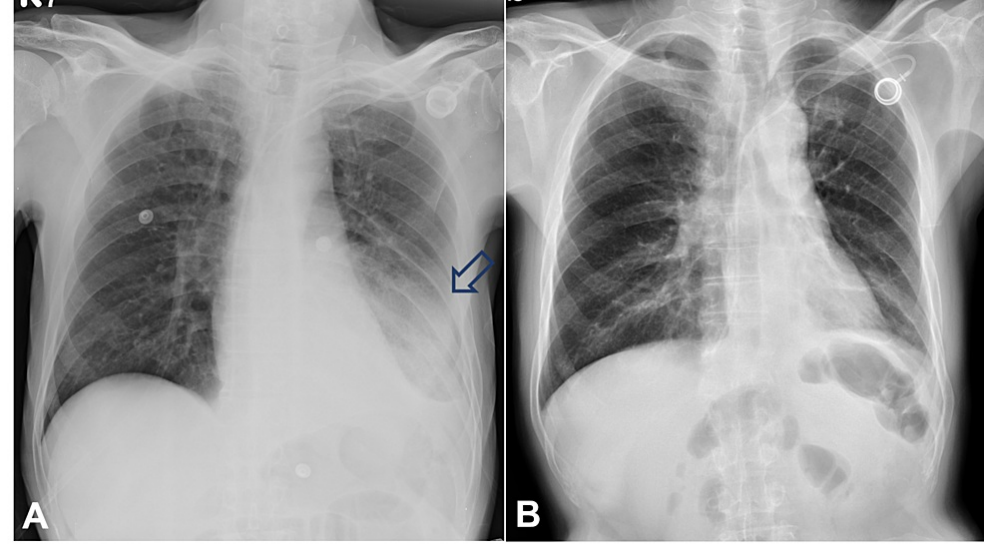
Chest X-ray films of the patient during treatment. A. Pneumonia in the left lower lobe (blue arrow) four days after the second cisplatin/paclitaxel chemotherapy (Jul. 18, 2020). B. Nearly complete resolution of the pulmonary metastasis and pneumonia eight days prior to the fourth course of cisplatin/gemcitabine chemotherapy (Jan. 6, 2021).
